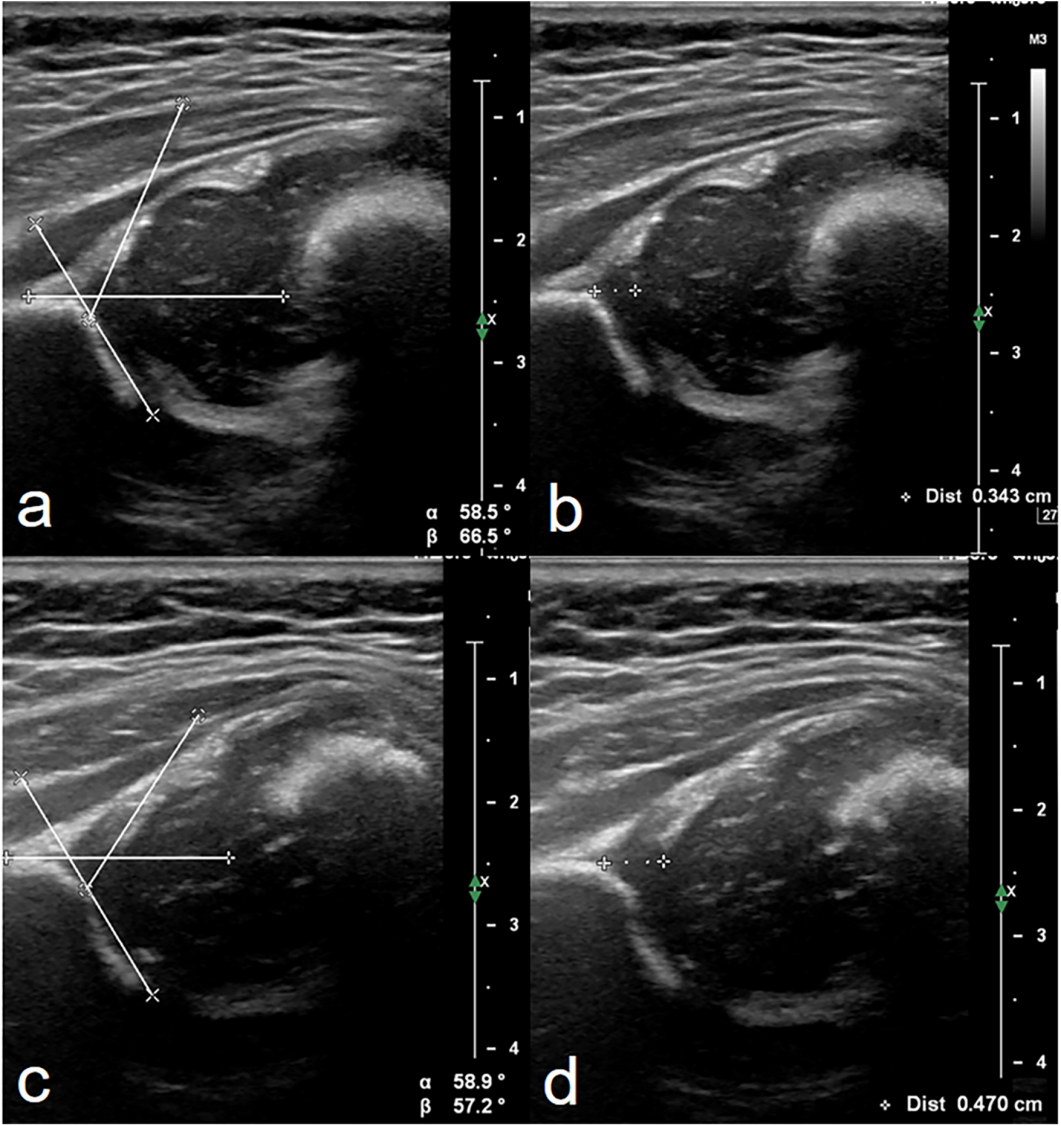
Measurement of alpha angle and the thickness of acetabular cartilage of hips in ultrasound images. Both were evaluated in the standard coronal plane described by Graf (10). Images (a,b) were from a 46-day-old female infant identified to be normal after a follow-up scan of hips 1 month later. Images (c,d) were from a 118-day-old female infant accepting treatment with the Pavlik harness for 1 month with a history of breech presentation. The same values of alpha angle (59°) were evaluated in these two patients. The thickness of acetabular cartilage in d was thicker than that in b. This provided more information to pediatric orthopedists in addition to alpha angle.
